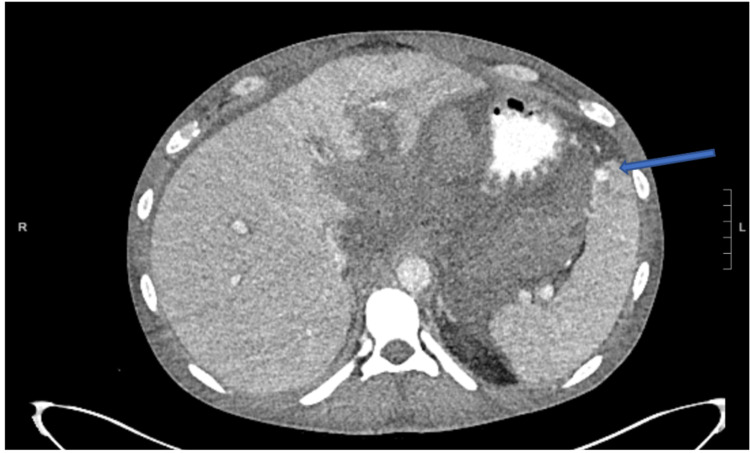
Contrast-enhanced axial CT of the abdomen demonstrating a focus of contrast opacification representing a pseudoaneurysm
